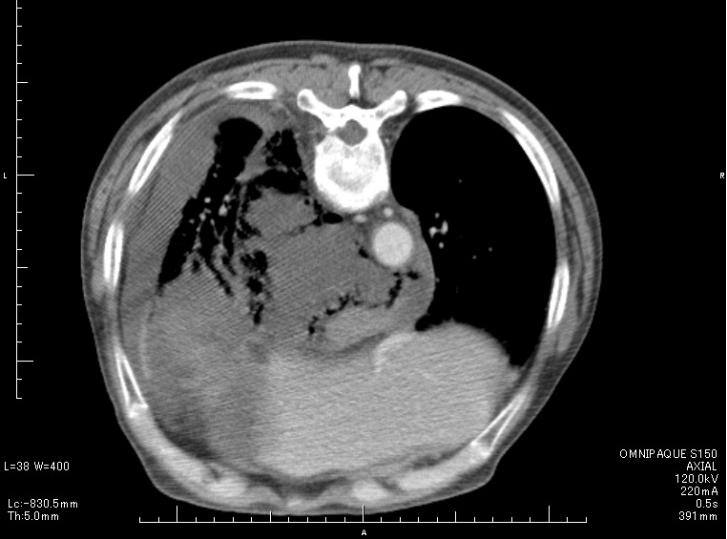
Chest CT. Large amounts of residual food and air were observed in the mediastinum, and pleural effusion was also present.
